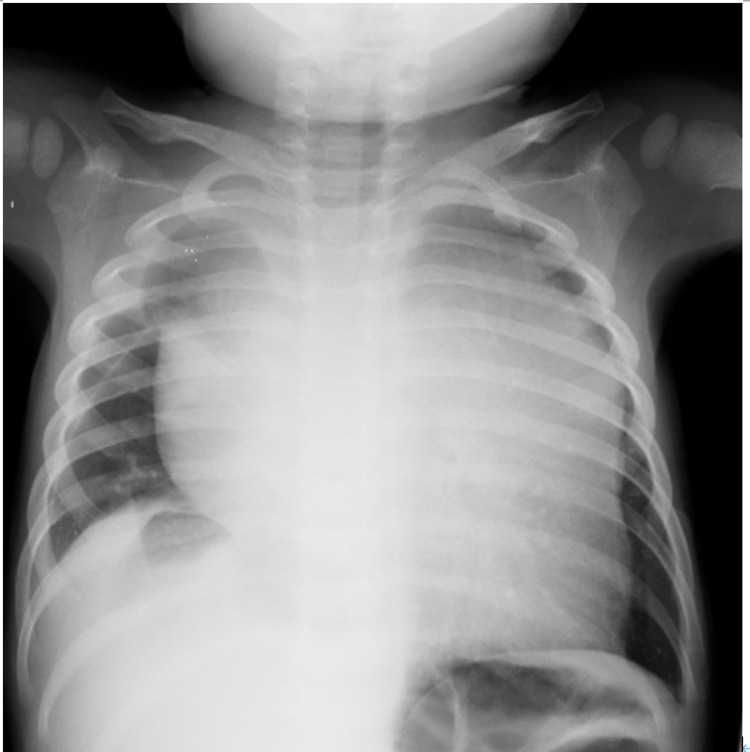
Chest X-ray Chest X-ray shows expanded mediastinal shadow. This is the first chest X-ray taken.
